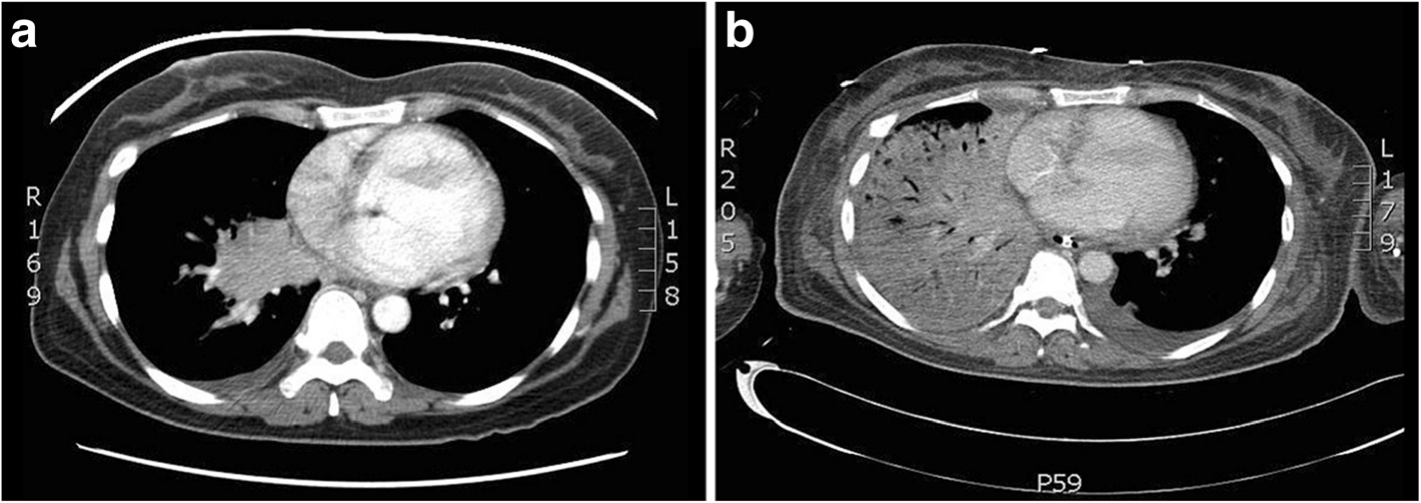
a Thoracic CT scan at presentation. Irregular mass impacting the right superior lobar bronchus. b Thoracic CT scan on the eighth day in the ICU. Extensive right pulmonary consolidation.
